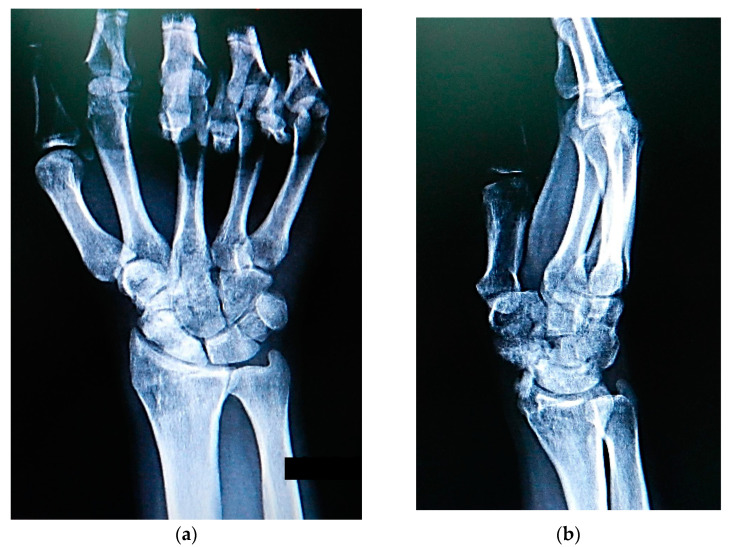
(a)Posteroanterior and (b) lateral X-rays revealing complete bony union of the scaphoid nonunion and calcification of the pronator quadratus muscle at 6th month postoperatively.
